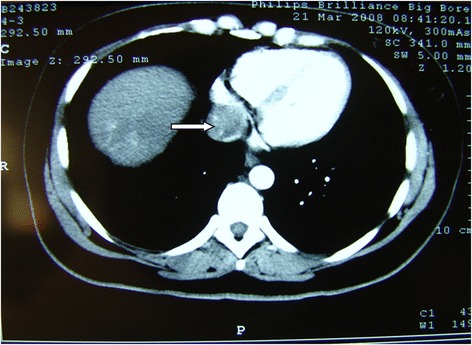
Enhanced CT arterial phase of a 30-year-old female showed filling-defect in IVC/RA (→)
